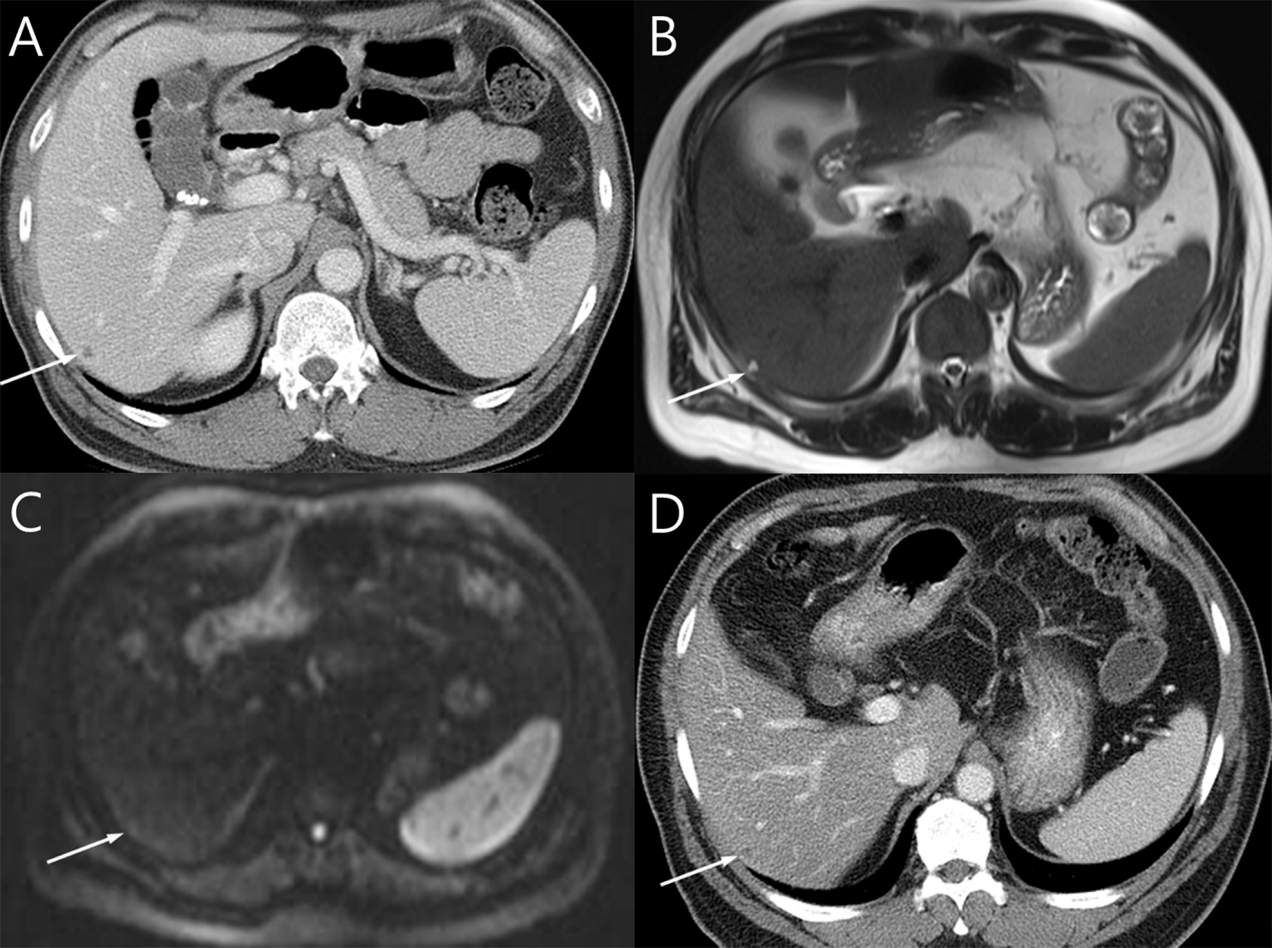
A 56-year-old male diagnosed with distal rectal cancer. (A) A 0.6 cm low attenuating nodule is noted in segment six of the liver on CT. It is difficult to determine whether it is a benign lesion or a small liver metastasis on CT. (B) The lesion shows high signal intensity on T2WI. (C) The nodule has high signal intensity on DWI (b = 50, 500 s/mm^2^) but iso-signal intensity on DWI (b = 900 s/mm^2^) without diffusion restriction. (D) The small nodule shows no interval change on follow-up CT obtained 2 years after initial CT.
